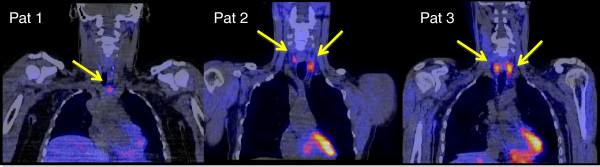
Coronal slices of fused 3-D-reconstructed SPECT/CT images of all three patients. Yellow arrows show the parathyroid adenomas in each image.
